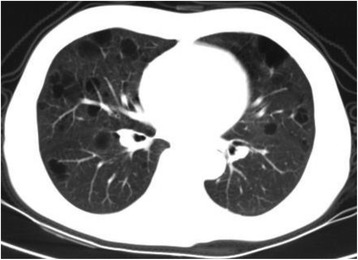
Thoracic high resolution computed tomography showing numerous well-defined thin-walled cysts
